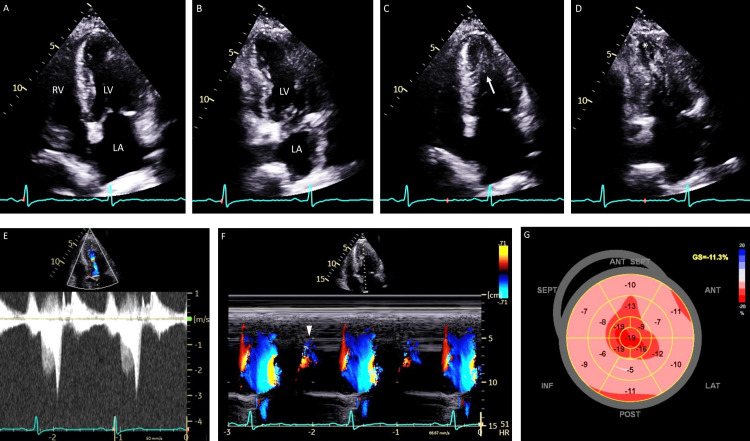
Echocardiography. Apical four- and two-chamber views at end-diastole (A and B) and end-systole (C and D) show mid-ventricular obstruction (C, arrow) with a suspicion of an apical chamber (D, asterisk). Doppler imaging shows a peak velocity of approximately 3.1 m/s in the mid-ventricle (E). Note an early diastolic flow from the apex to the base of the left ventricle during a period of isovolumic relaxation to diastole or paradoxic jet flow (F, arrow). Two-dimensional speckle-tracking echocardiography reveals a global longitudinal strain (GS) of -11.3% with an apical sparing pattern (G). LA: left atrium; LV: left ventricle; RV: right ventricle; HR: heart rate; SEPT: septal; INF: inferior; POST: posterior; LAT: lateral; ANT: anterior; ANT SEPT: anterior-septal
